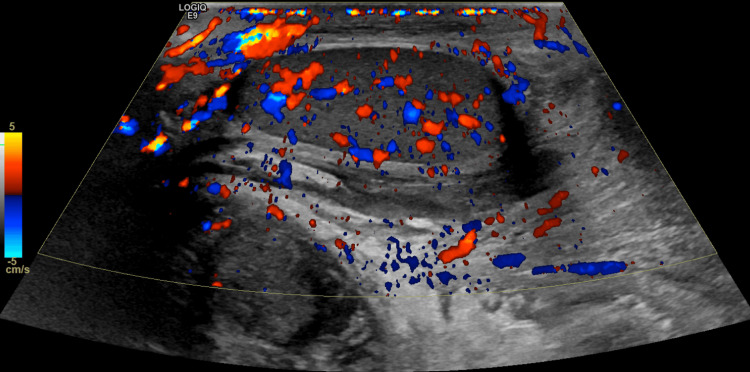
Ultrasonography of the left testis showing hypervascularity, suggesting severe epididymoorchitis.
